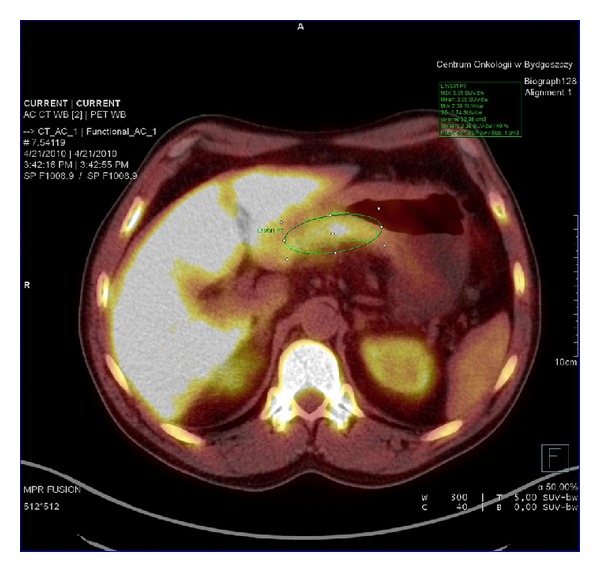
^18^FLT-PET in patient with mucinous adenocarcinoma. SUV_max⁡_ = 5.9.
